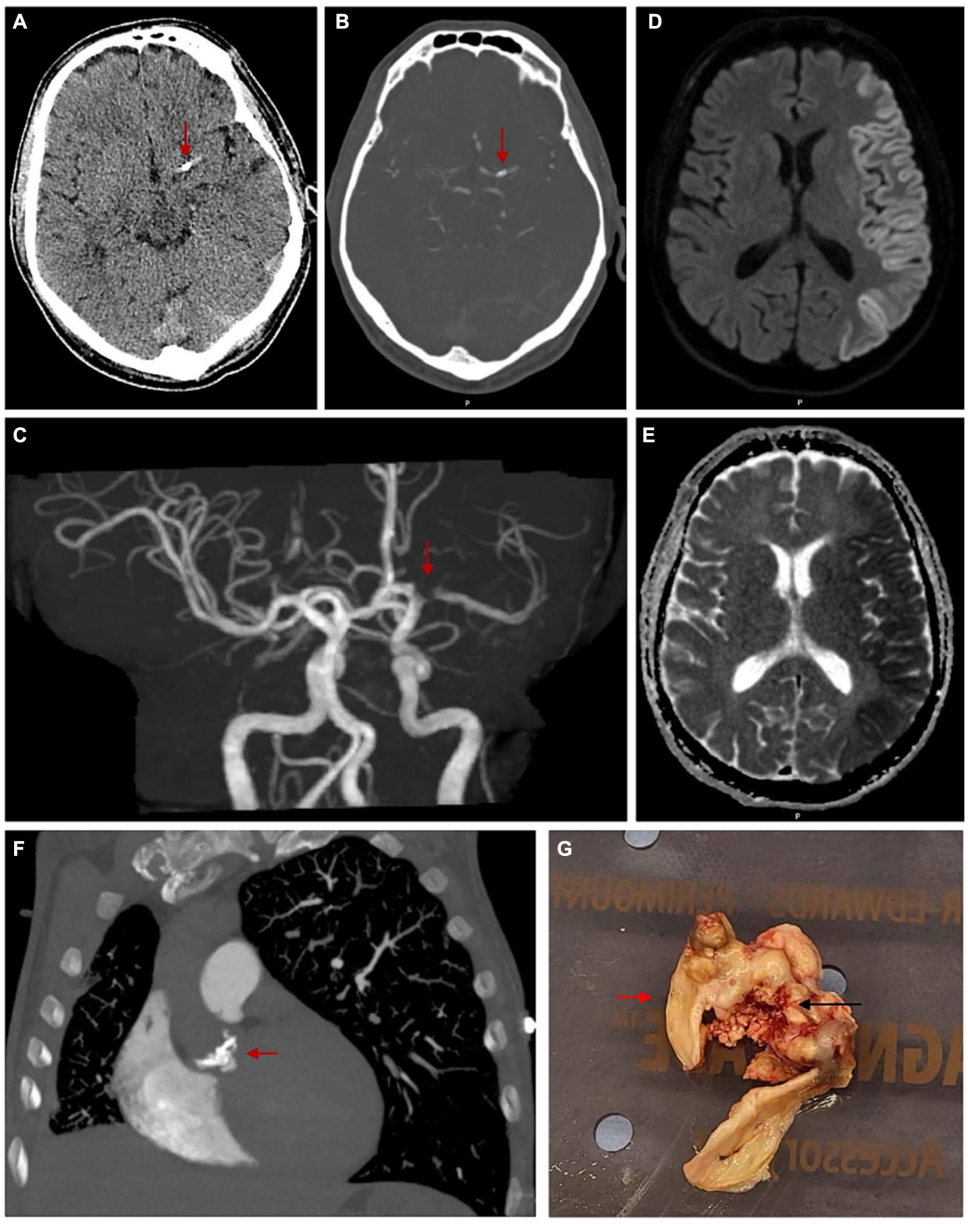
Images demonstrating a calcified cerebral embolism in the M1 segment of left MCA, left hemispheric cerebral cortex diffusion restriction and nodular calcification of bicuspid aortic valve leaflets. (A) Axial view of unenhanced CT brain with red arrow marking a calcified focus measuring 6 mm in the vicinity of proximal left MCA. (B) Axial view of CT brain angiogram with red arrow marking a calcified focus involving the proximal M1 segment of left MCA. (C) 3D reconstruction of CT brain angiogram with red arrow marking a filling defect involving the M1 segment of left MCA, corresponding with site of calcific cerebral embolism. (D) MRI brain diffusion-weighted images in axial view demonstrating left hemispheric cortical hyperintensity or ‘Cortical Ribbon Sign’. (E) Corresponding MRI brain apparent diffusion coefficient images in axial view demonstrating left hemispheric cortical hypointensity. (F) CT pulmonary angiogram in coronal view with red arrow marking multiple densities/calcifications over the bicuspid aortic valve. (G) Macroscopic photo taken intra-operatively with red arrow marking normal bicuspid aortic valve and black arrow marking dystrophic calcification over bicuspid aortic valve.
